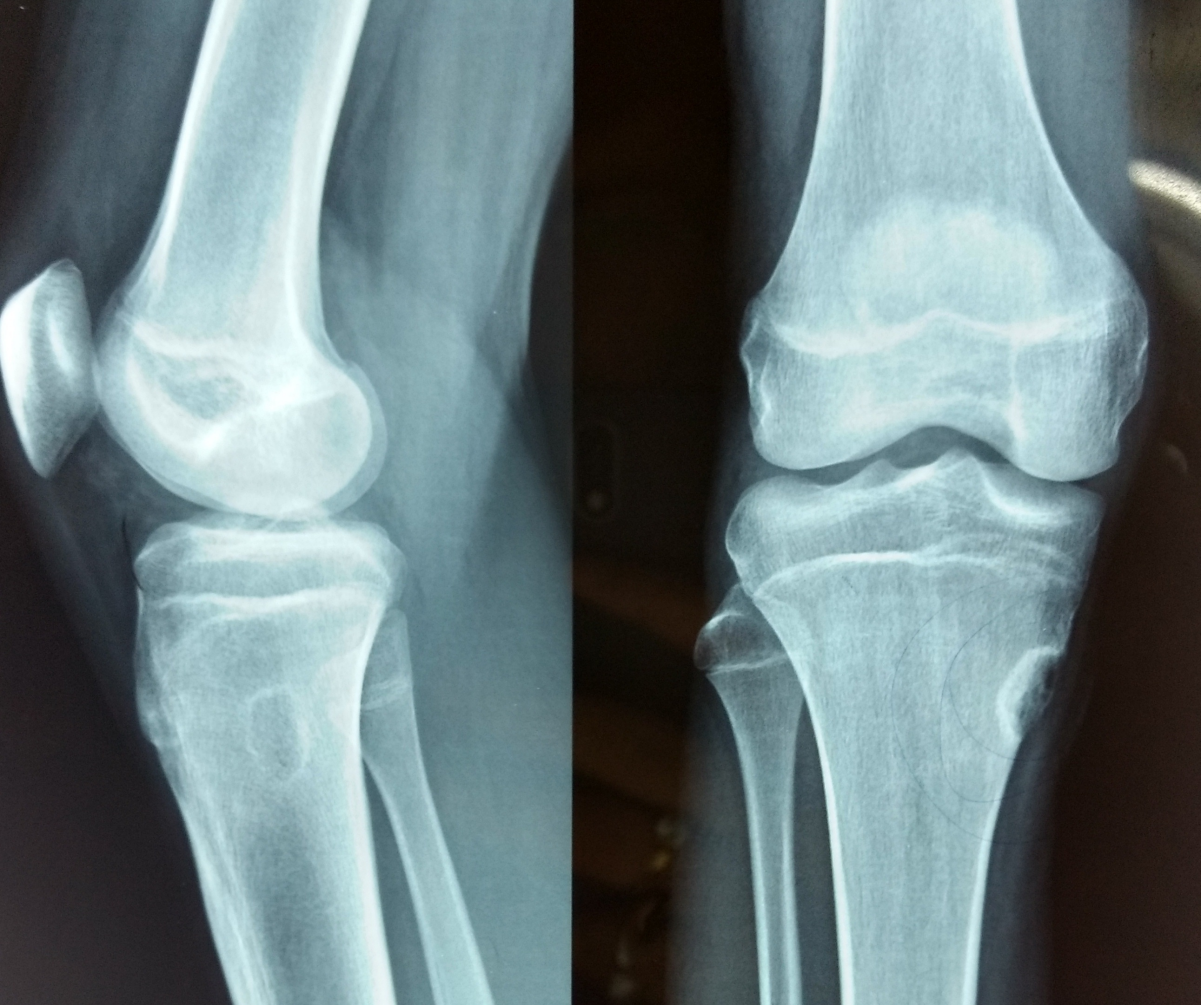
Pre-op radiograph (anteroposterior and lateral views) The images show the lesion in the metaphyseal area of the tibia on the medial aspect.
